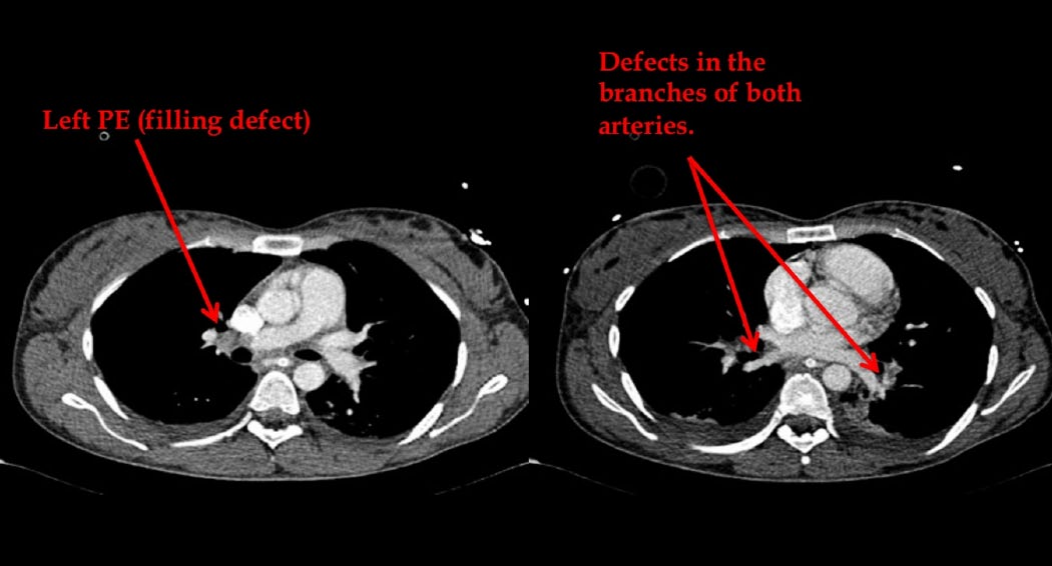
Slides from CT chest that show the left PE and defects in the branches of both pulmonary arteries.
